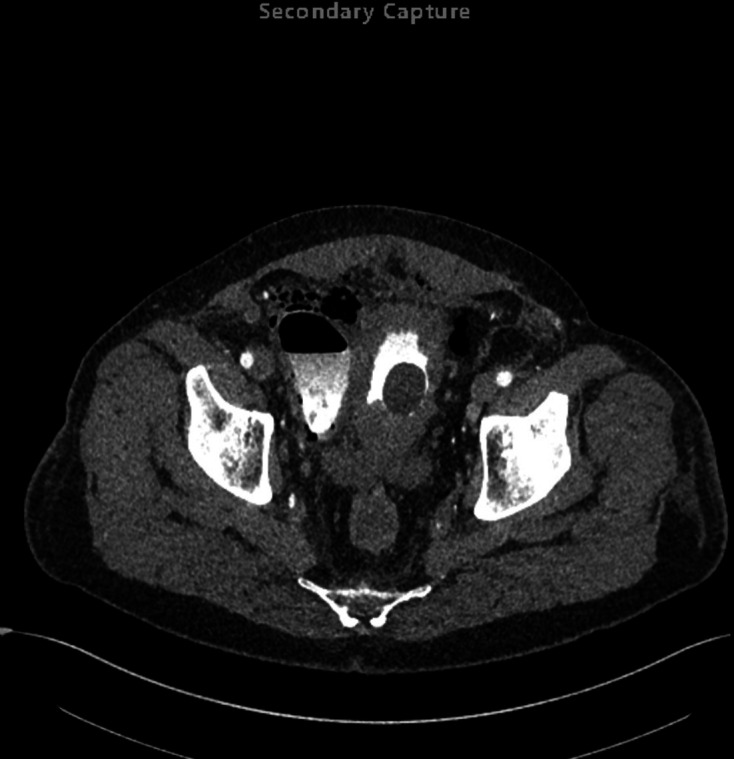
First axial delayed phase CT (Day 3 of admission), on soft tissue window
